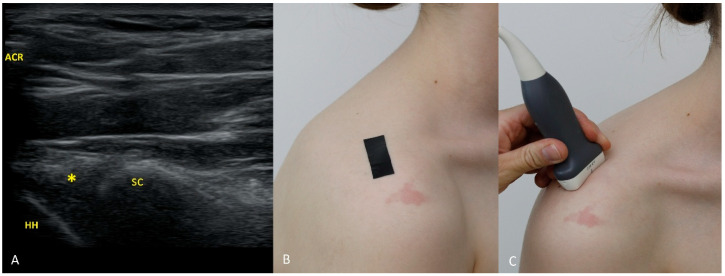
The 12 o’clock position. For the 12 o’clock view, place the transducer vertically over the supraspinatus fossa, in the soft spot anterior to the scapular spine, posterior to the clavicle, and medial to the acromion, with the transducer partly overlapping the medial acromial edge. Medial and deep to the acromion, the supraspinatus muscle is seen; deeper still, the outline of the scapula and the region of the suprascapular notch; more laterally, the bony glenoid rim. The labrum attaching to the glenoid is usually visible without additional maneuvers. Occasionally, however, the labrum lies in the acoustic shadow of the acromion, depending on minor anatomical variations. If the labrum is not visible after initial transducer placement, we recommend remaining with the linear transducer and activating trapezoidal imaging, or switching to a convex transducer. With trapezoidal imaging, ultrasound waves propagate not only perpendicularly to the transducer face but also at an angle (e.g., ~15°), which allows one to “peek” under the acromion. Note that in the central portion of the field the beams travel almost exclusively perpendicular to the transducer face; the benefit of the oblique beam occurs when the structure of interest (here, the labrum) is positioned near the edge of the field. A similar effect can be obtained with a convex transducer by placing the structure near the field margin. Using these techniques not only enables visualization of the superior labrum but often also of a portion of the long head of the biceps tendon.
